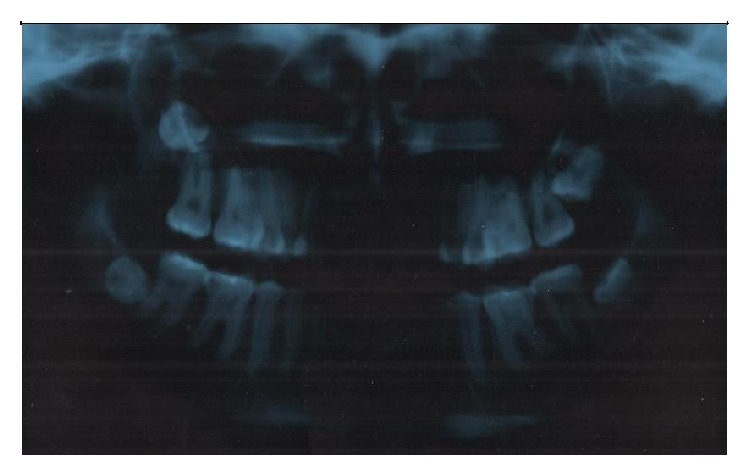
X-ray with the DU 18 into the maxillary sinus.
